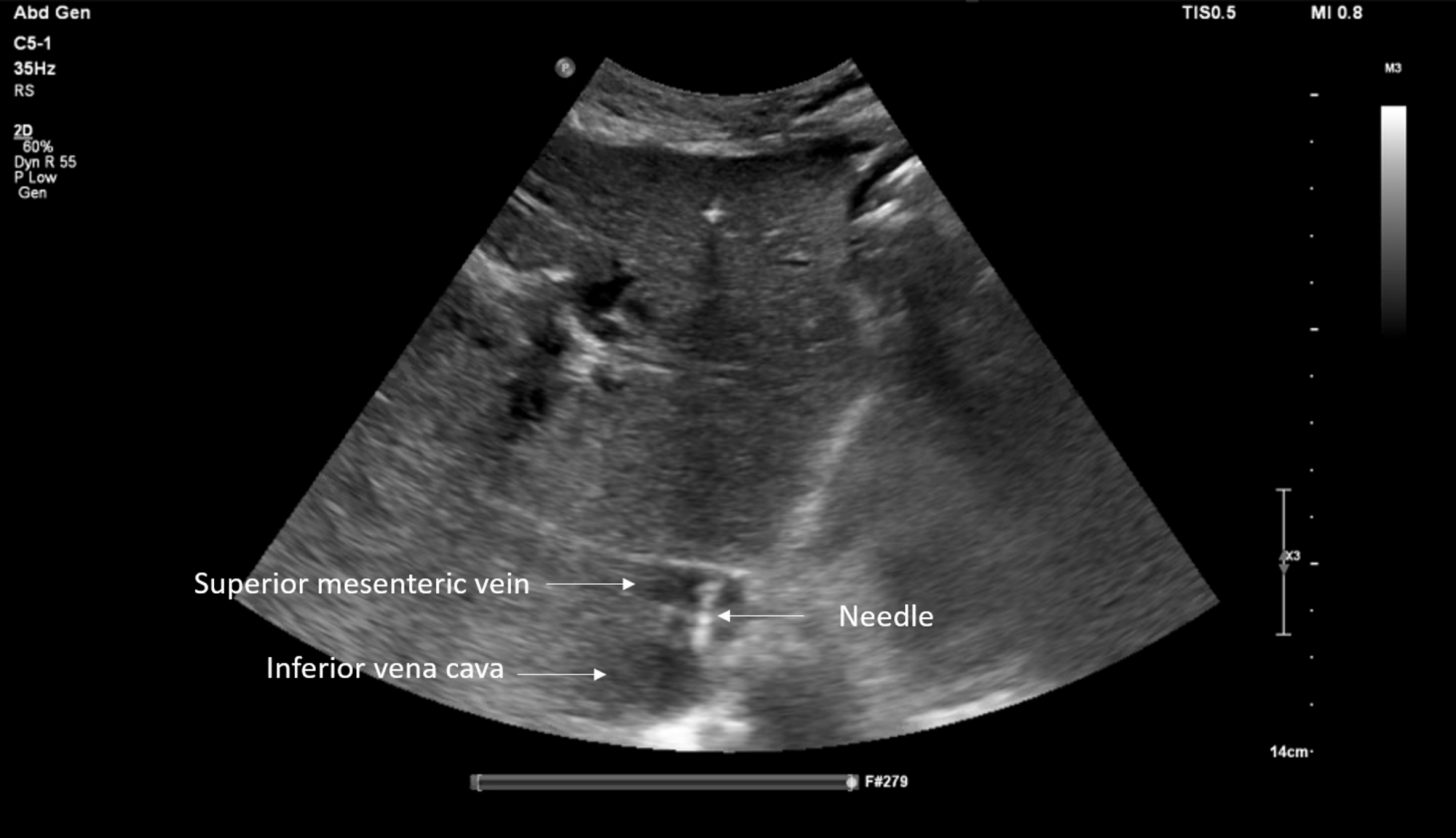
Ultrasonographic finding shows the needle catheter system was passed from the inferior vena cava to the cavernous portal vein branch
